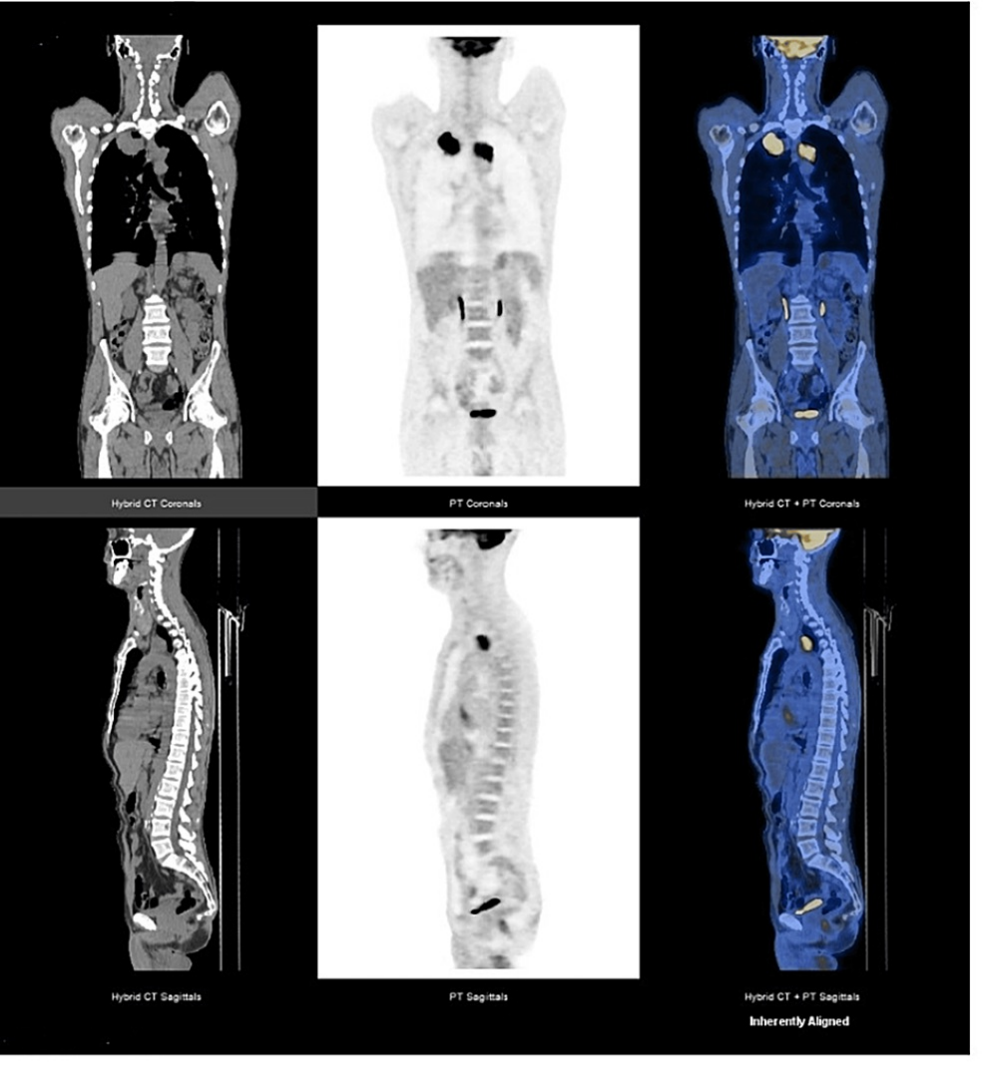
Staging FDG/PET-CT performed in May 2020
